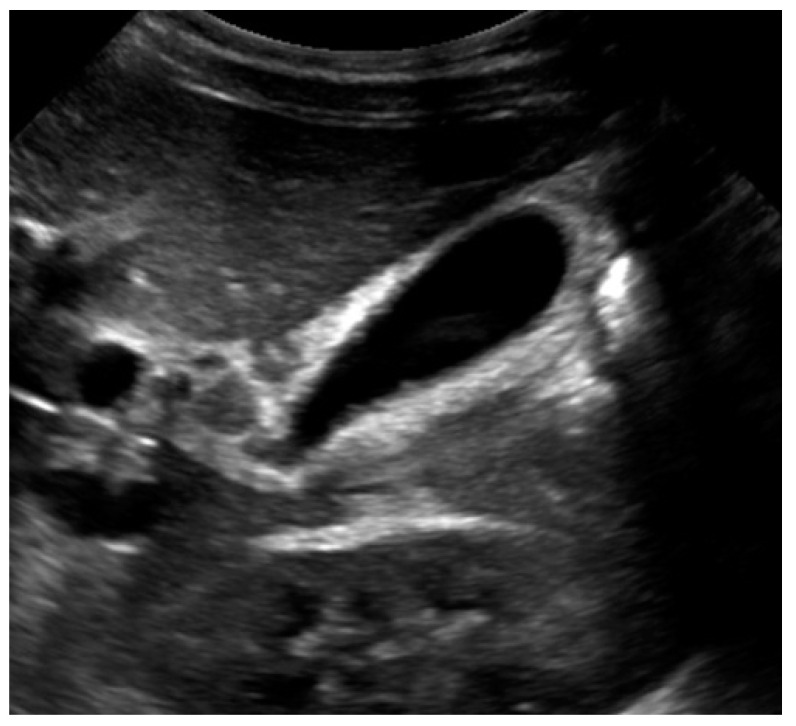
Abdominal ultrasonography showing a thickening of the gallbladder wall of 4.5 mm and pericholecystic edema.
